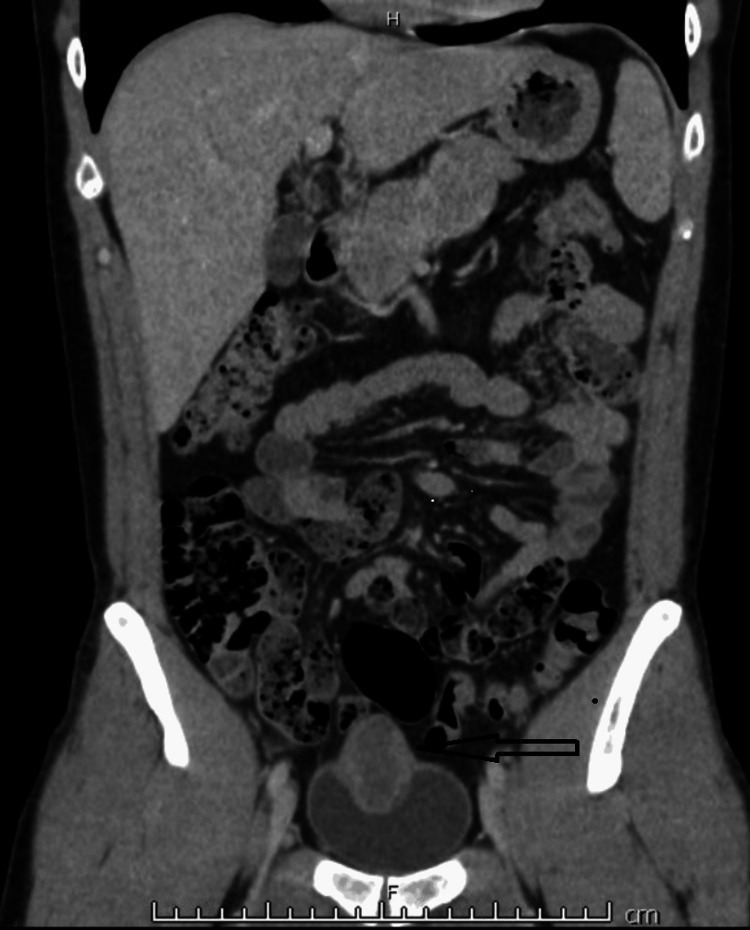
Coronal section of CT abdomen/pelvis demonstrating the apical bladder mass
